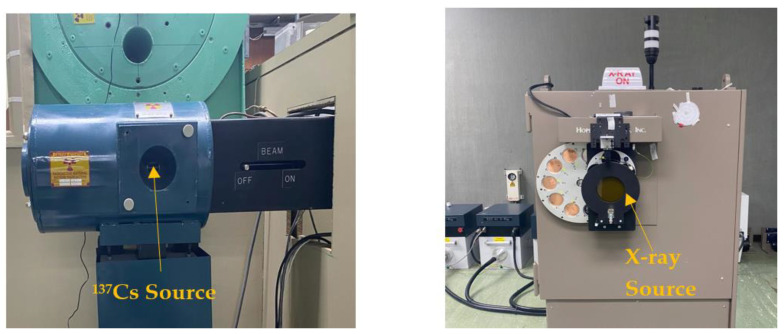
KFSH&RC-SSDL 450 Ci 137Cs and X-ray sources.
